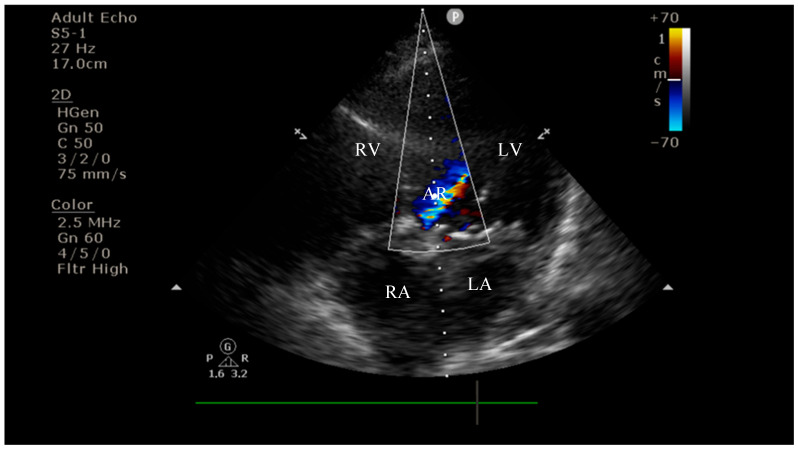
Apical four-chamber view on admission demonstrating moderate aortic regurgitation; LV—left ventricle; RV—right ventricle; LA—left atrium; RA—right atrium; AR—aortic regurgitation jet.
